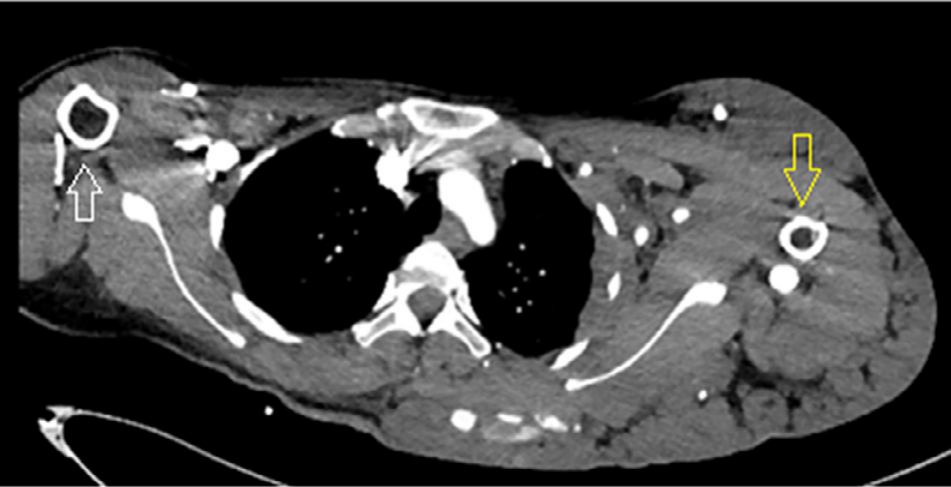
Computed tomography showing the diameter of the left humerus (white arrow) smaller than the right (yellow arrow). (Color version of figure is available online.)
